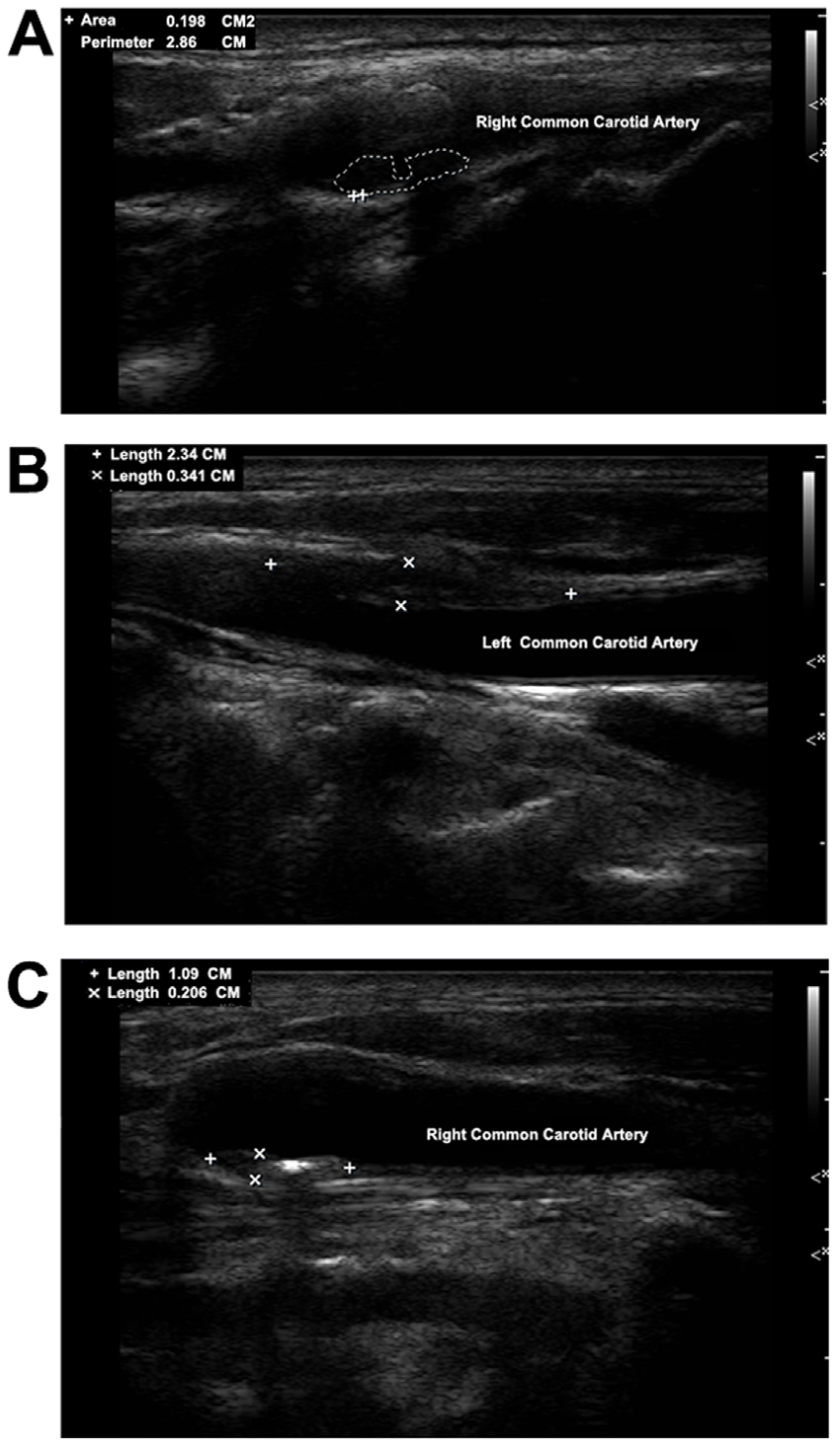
Representative ultrasound images for different types of advanced carotid plaques in study participants; (A). ulcerated plaque; (B). plaque with low-level echo; (C). plaque with heterogeneous echo.
